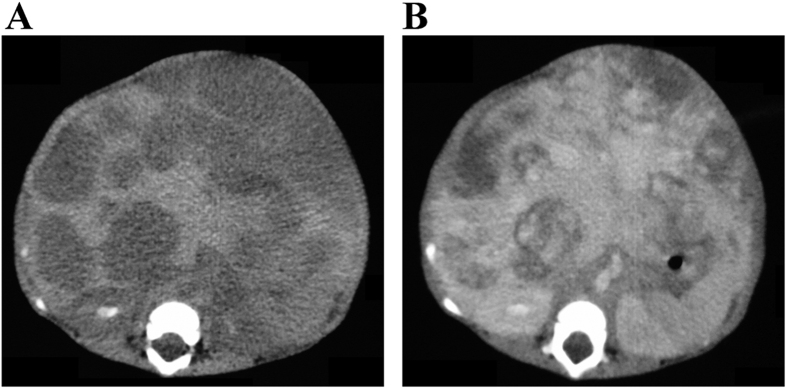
MHH in a 4.5-month-old boy (Patient #2 in Table 2). (A) An axial unenhanced CT scan showed multiple hypodense hepatic masses ranging from 1.0 to 4.0 in diameter. (B) An axial enhanced CT scan showed an enhancement of the liver masses. Some of the masses showed confluence.
